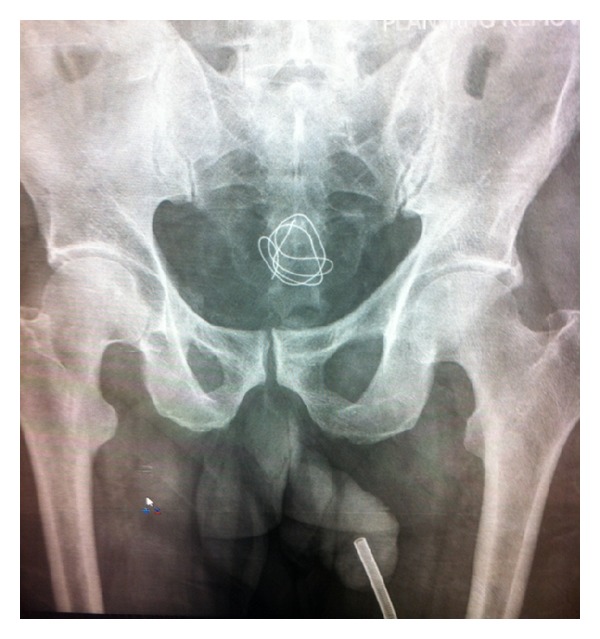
Plain film demonstrating antenna in penile urethra and radiopaque foreign body within the pelvis. Of note the fishing line with attached beads was not visible.
